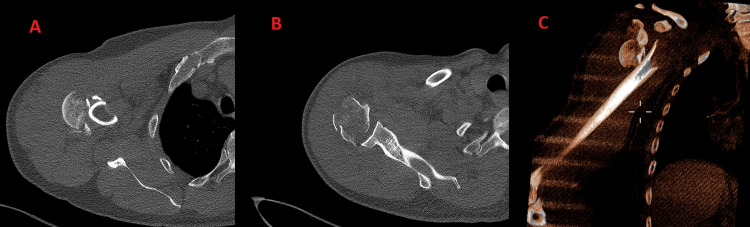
CT scan views of the right shoulder. A: The first fragment of the split humeral head. B: The second fragment of the split humeral head, positioned lateral to the glenoid fossa. C: Volume rendering view (VR view) from the CT scan of the right shoulder, demonstrating the comminution of the fracture.
